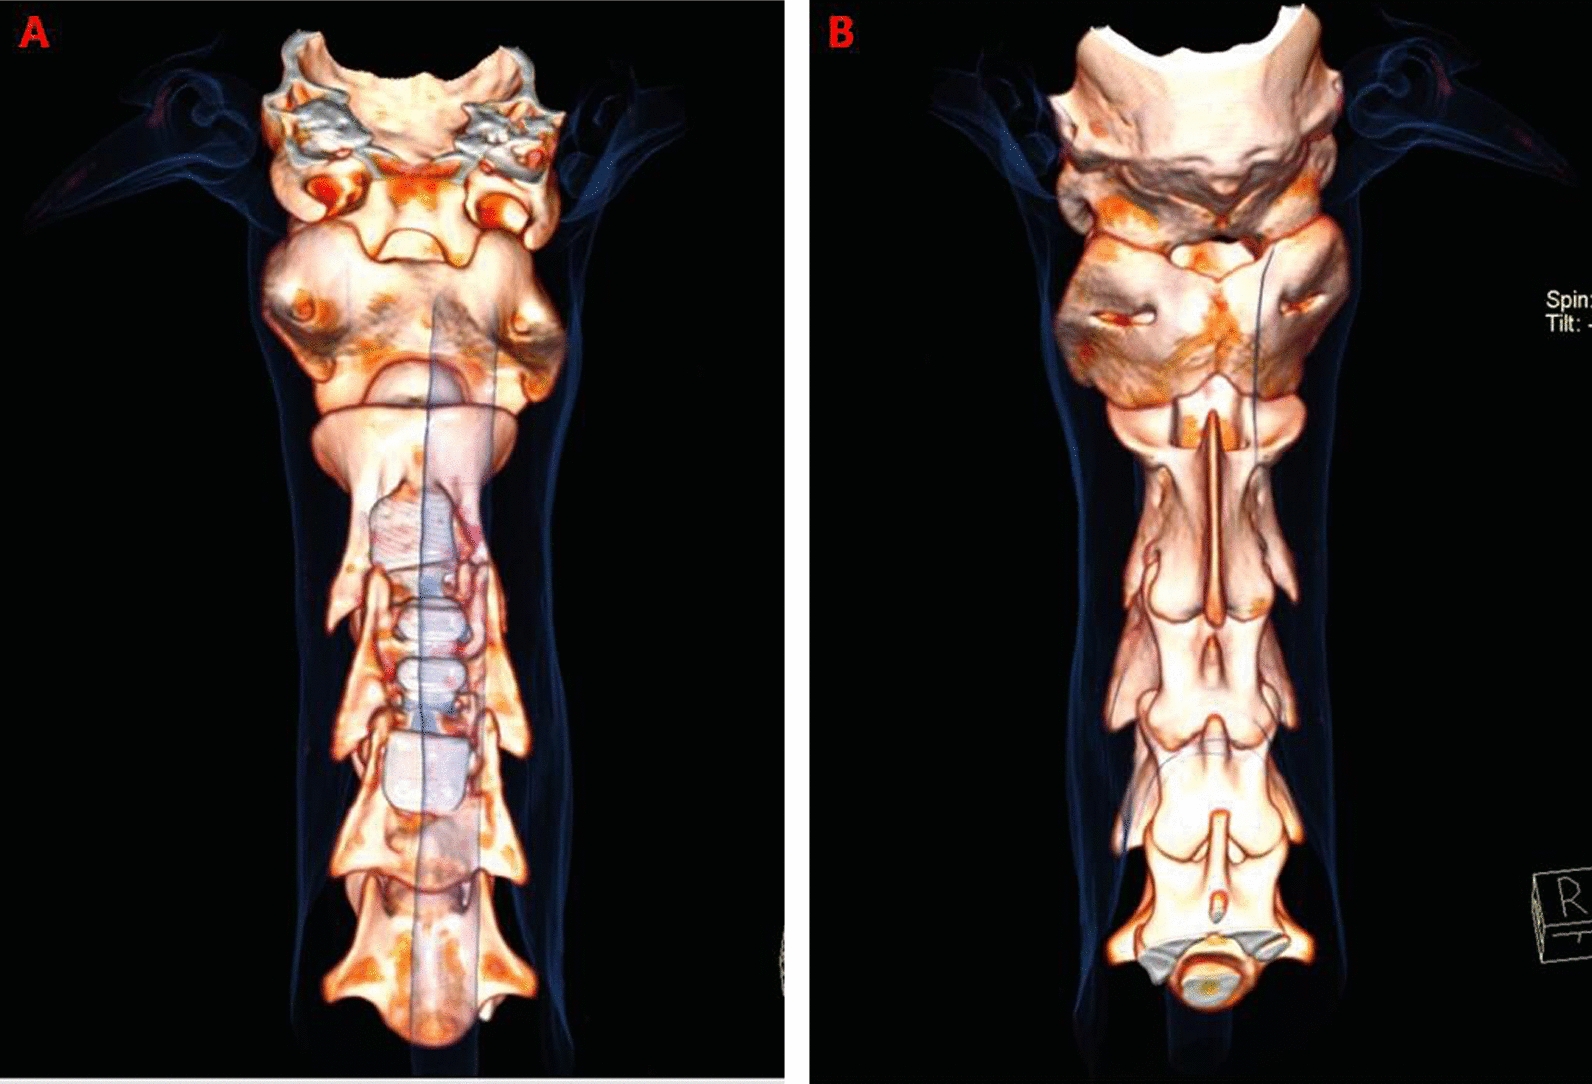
3D CT reconstruction 3 months after surgery. A, Front view. B, Back view
